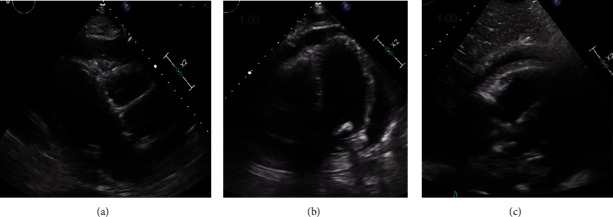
Parasternal long (a), apical 4-chamber view (b), and subxiphoid view (c) via echocardiography of expanding pericardial effusion on hospital day 7. No right atrial or right ventricular collapse or respiration variation in mitral or tricuspid inflow was seen. The inferior vena cava (IVC) was enlarged without collapse.
